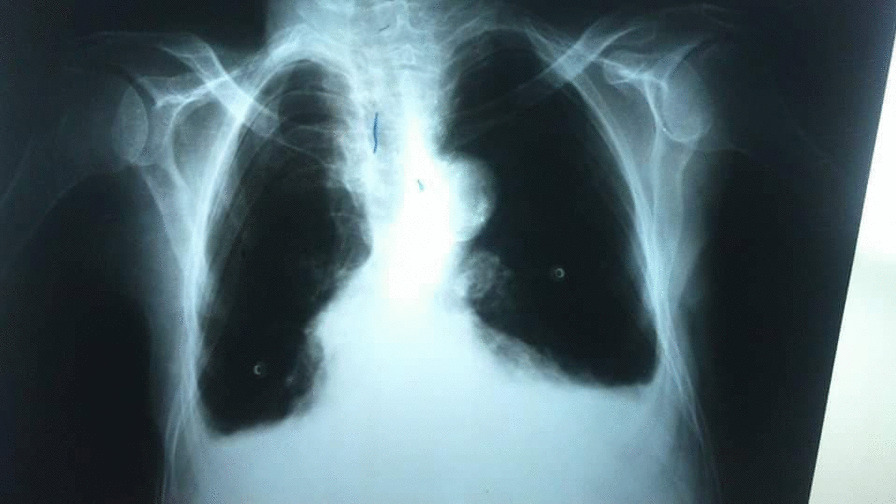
Posterior-Anterior erect chest x-ray showing a bilateral pleural effusion
